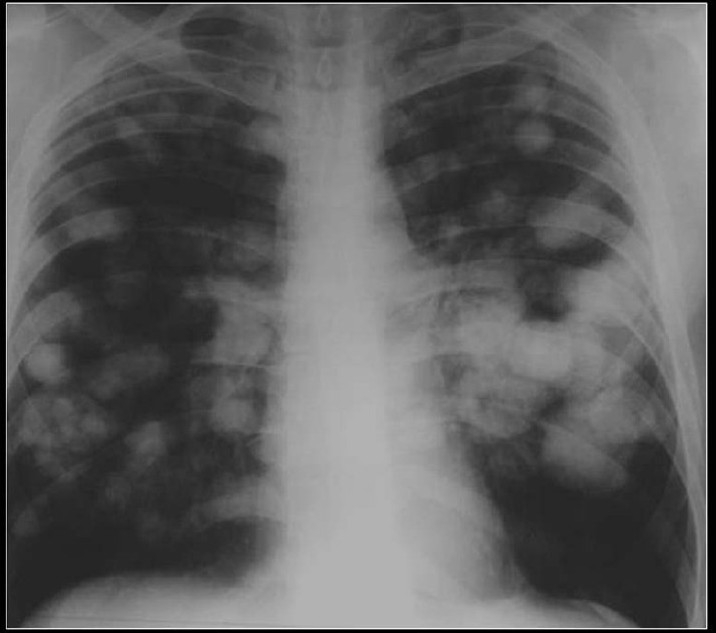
A chest radiograph showing calcified metastases from an osteogenic sarcoma. Note that the density of the tumors and the skeletal tissues is similar
